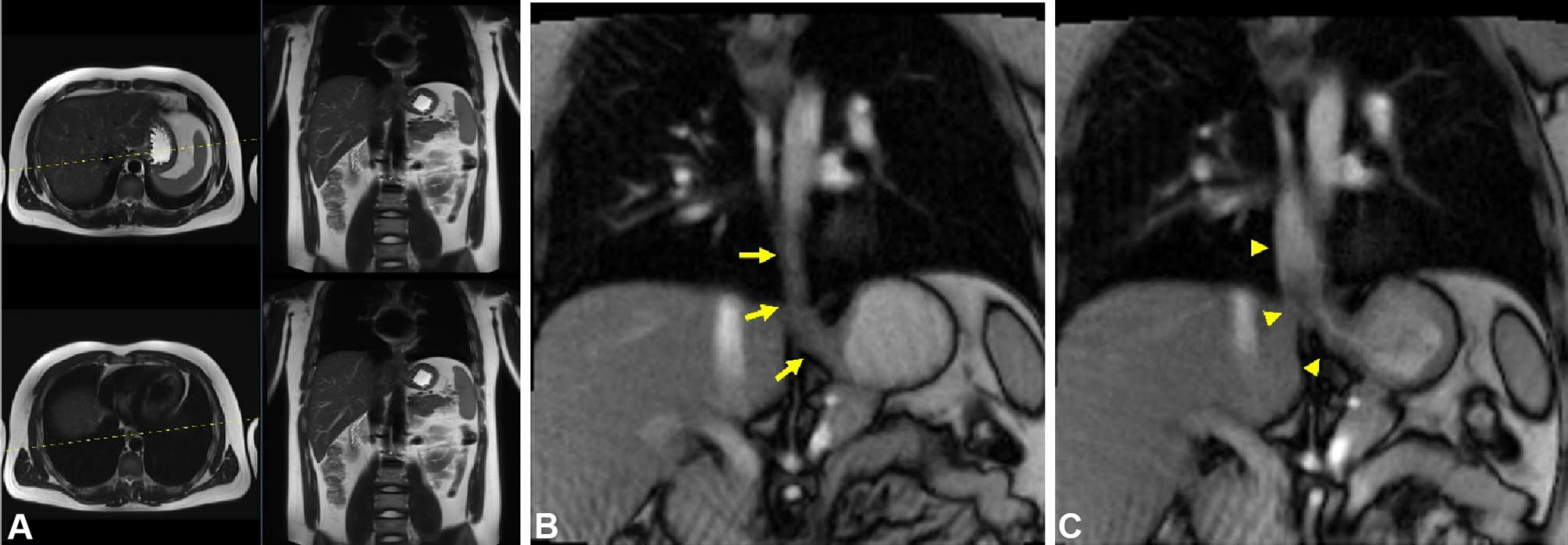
Definition of coronal real-time MRI of EGJ. (A) Axial and coronal HASTE planning images for coronal real-time MRI of EGJ. (B, C) Real-time MRI of the empty esophagus before bolus arrival (yellow arrows) and during bolus transit (yellow arrowheads).
